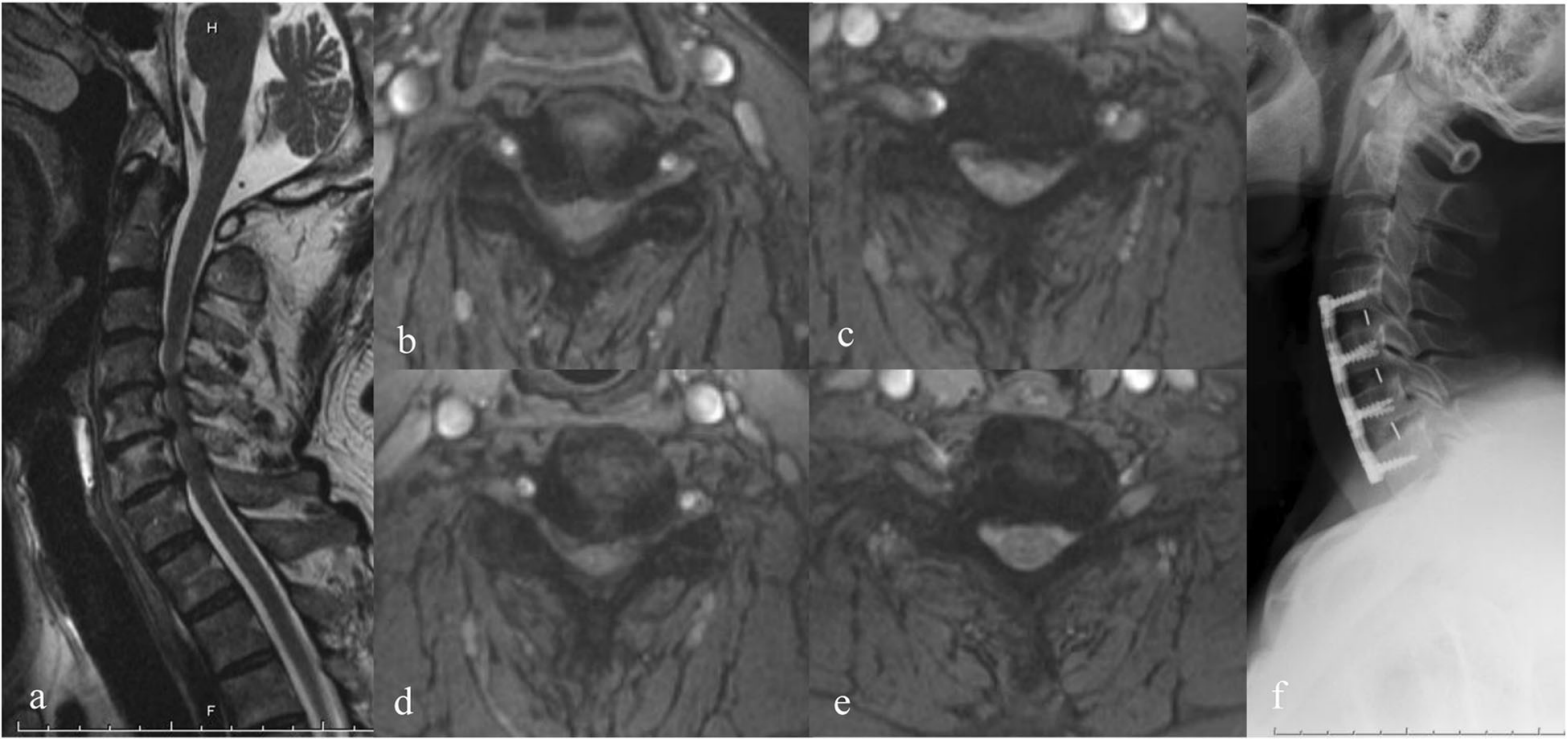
ACDF treatment of MCSM. a A 65-year-old male patient was diagnosed as MCSM with typical symptoms of superior motor neurons compression. T2-weighted sagittal MRI showed spinal cord compression in C4/5, C5/6, C6/7. b–e C4/5, C5/6, C5/6, C6/7 spinal cord compression on T2-weighted axial MRI. f Multi-level ACDF was performed to release the compression. Lateral X-ray of cervical spine was taken at 2-year follow-up visit and sagittal parameters were corrected appropriately
